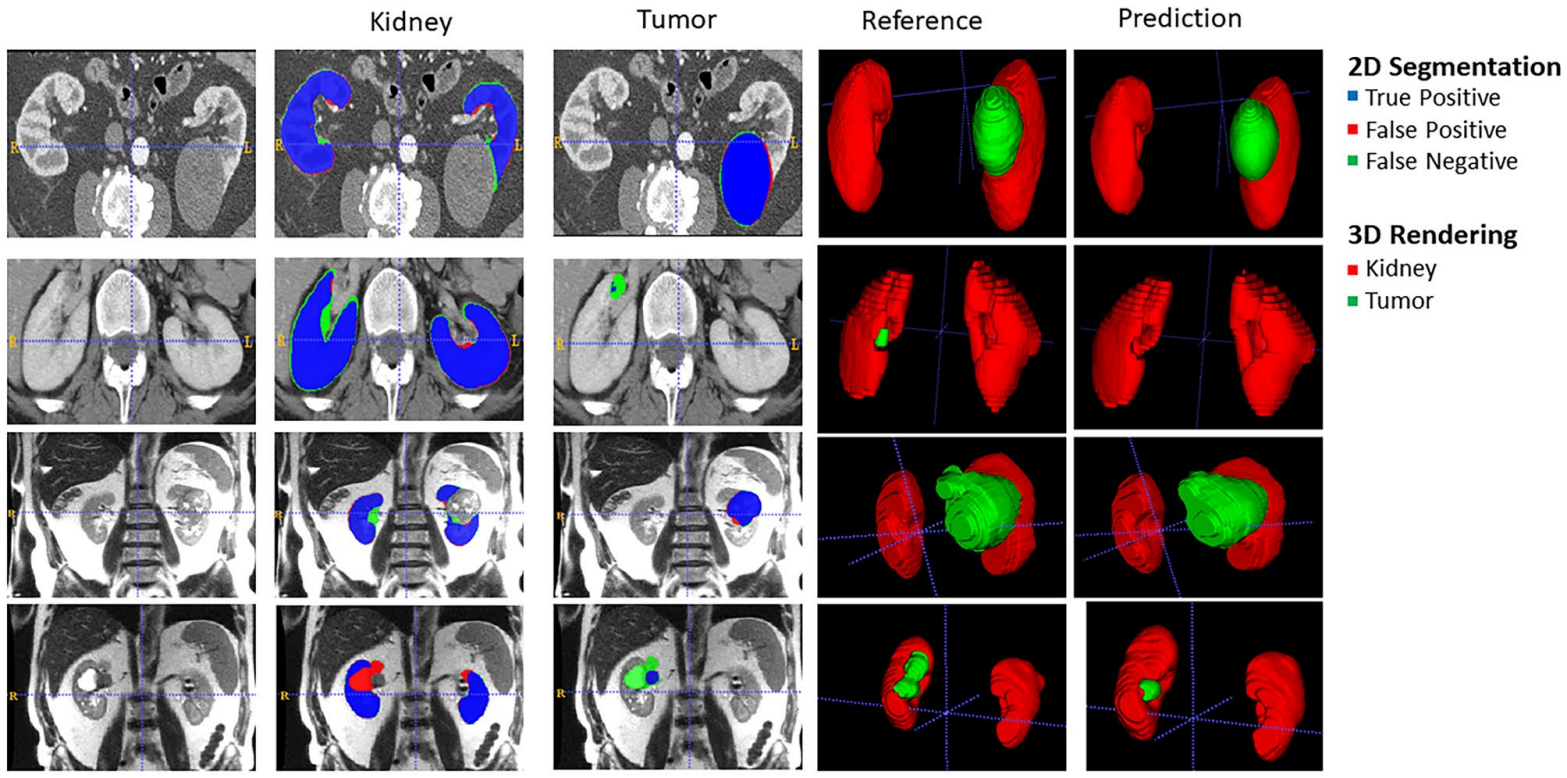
Test metrics of final ensemble 300-dataset size model predictions for each testing set example colored by reference segmentation volume. a CT kidney. b CT tumor. c MR kidney. d MR tumor
